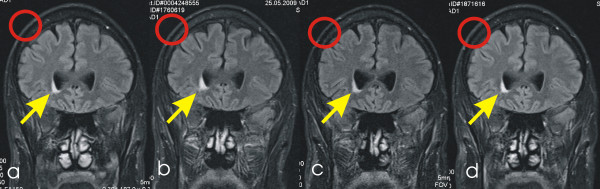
A lesion followed over time. Coronal fluid attenuated inversion recovery (FLAIR) image. The arrow points to a small periventricular lesion. The lesion shows no difference during the time course. However, the thickness of the subcutaneous fat layer (see red circle) shows a marked decrease from (A-C) and recovery at (D). (A) Timepoint 1 before the TransEurope-FootRace 2009 (TEFR09) in April 2009; (B) timepoint 2 during the race at 2,326 km; (C) timepoint 3 during the race at 4,005 km; (D) timepoint 4 at follow-up 8 months after the race.
